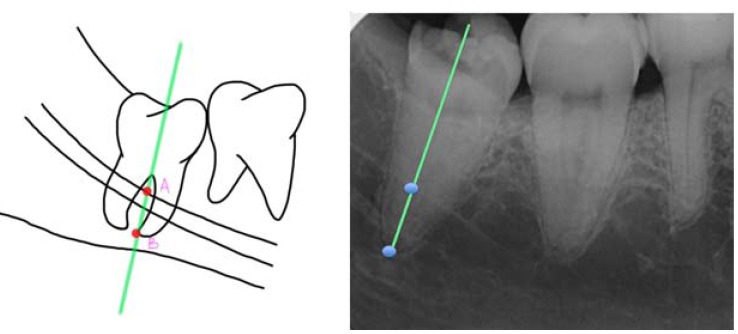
a) Calculation of the root migration through the distance between point A (superior cortical of the mandibular canal) and point B (apical point of lower third molar). Compilation prepared by the authors, based on the technique proposed by Leung *et al*. (15). (b) Example in the form of a radiographic image.
